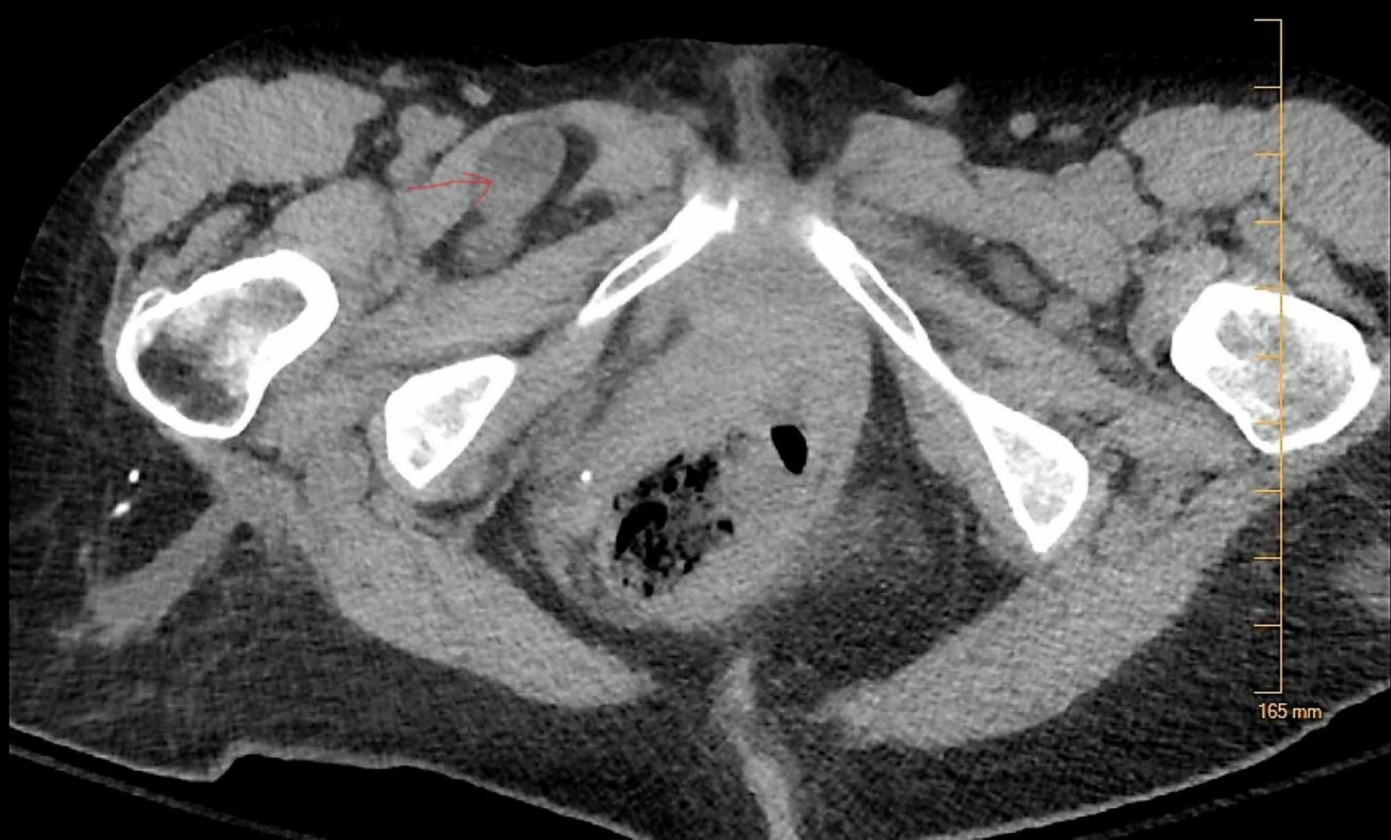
CT scan of the abdomen and pelvis showing the Right Obturator Hernia (Axial view).
